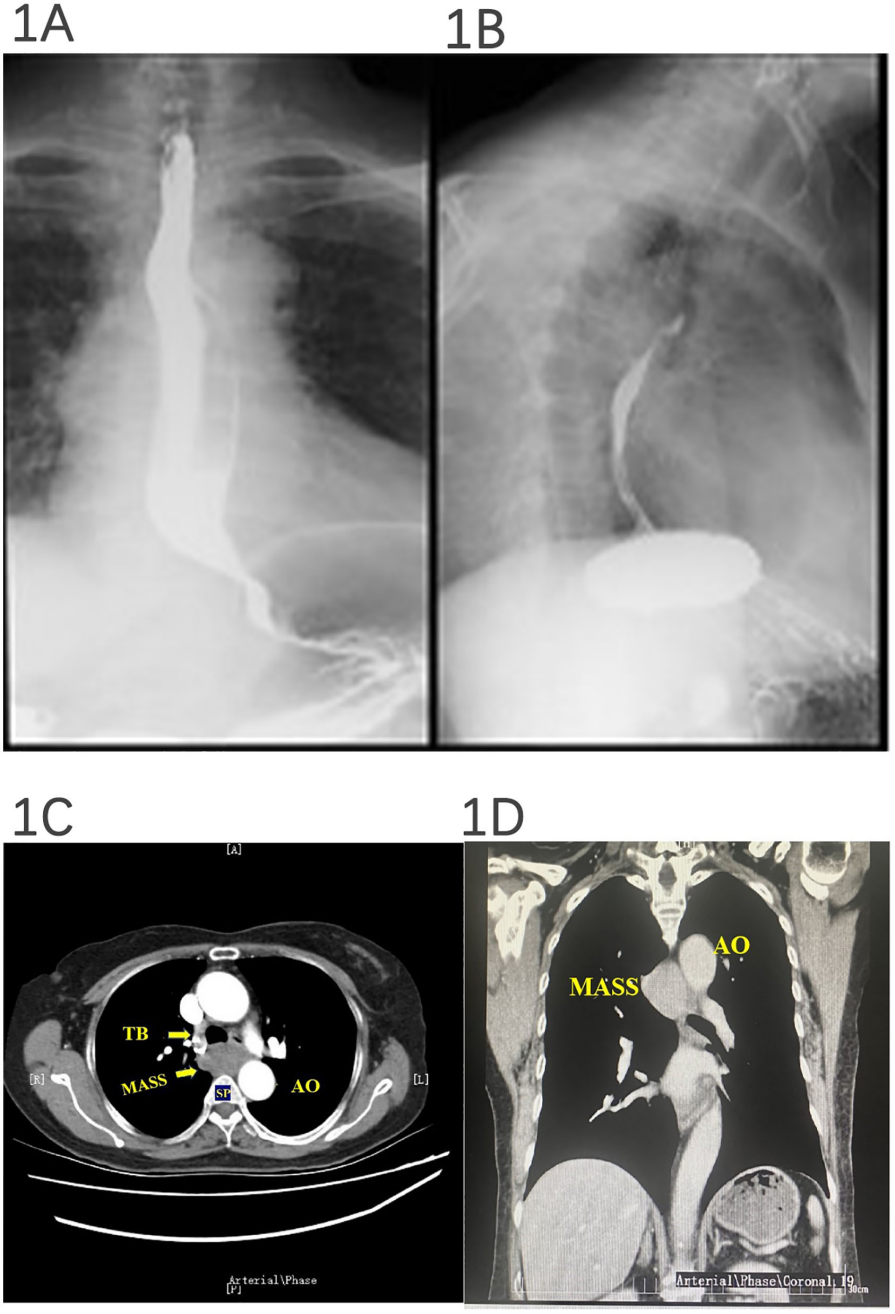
Barium meal fluoroscopy and esophageal and thoracic enhanced CT. The esophageal mucosa was smooth, barium could pass through smoothly, and peristalsis was seen, but the esophagus under the aortic arch was partially arc-shaped under pressure (A, B). (A) Esophageal luminal compression. (B) Azygos vein aneurysm. A posterior mediastinal mass was detected, and a subsequent contrast CT of the thorax revealed a 3.5 × 3.74 × 1.4-cm hypodense posterior mediastinal mass without enhanced signal that was located at the level of thoracic vertebrae 4–6, behind the trachea, under the carina, and in front of the esophagus (C, D).
